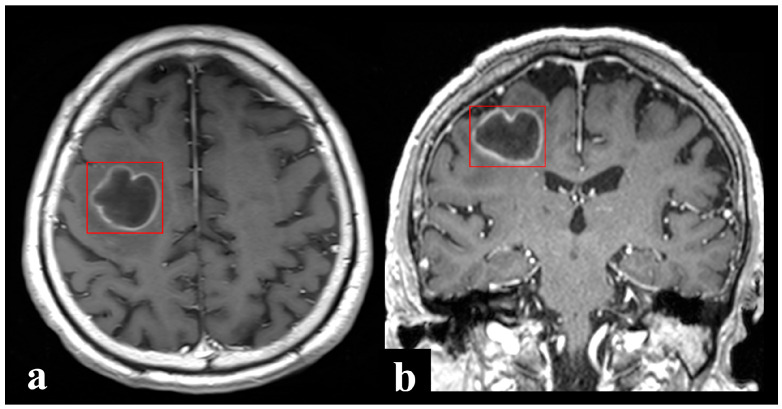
Depiction of bound box annotations of a right frontal ring-enhancing pyogenic brain abscess on (a) axial 2D and (b) coronal 3D post-contrast T1-weighted MRI images. [original image].
